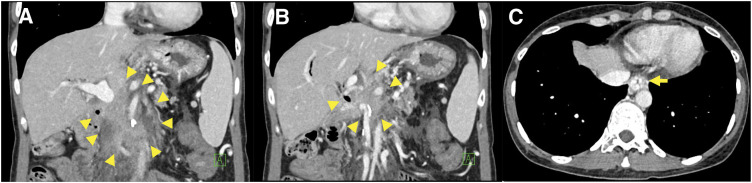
Enhanced CT at the time of hemorrhage. (A, B) Inflammatory changes were observed around the PV, along with severe stenosis of the PV and obstruction of the SV (arrowheads). (C) The development of esophageal varices was noted (arrow). PV, portal vein; SV, splenic vein
